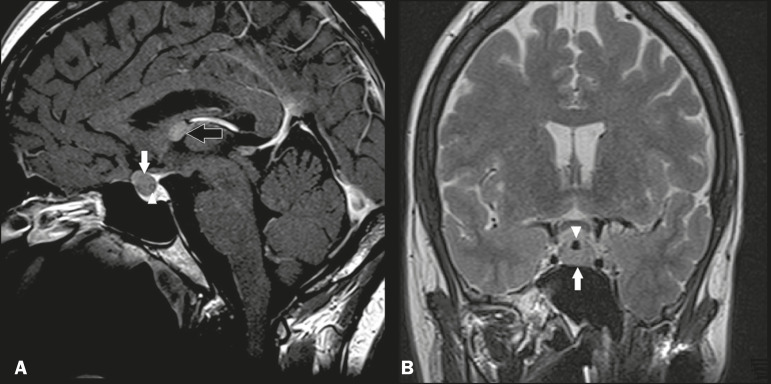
Rathke’s cleft cyst. Contrast-enhanced sagittal T1-weighted MRI (A) and coronal T2-weighted MRI (B), showing an expansile cystic lesion extending slightly into the suprasellar region, without contrast enhancement (white arrow). Note the small intracystic nodule (arrowhead), as well as the colloid cyst in the third ventricle (black arrow).
